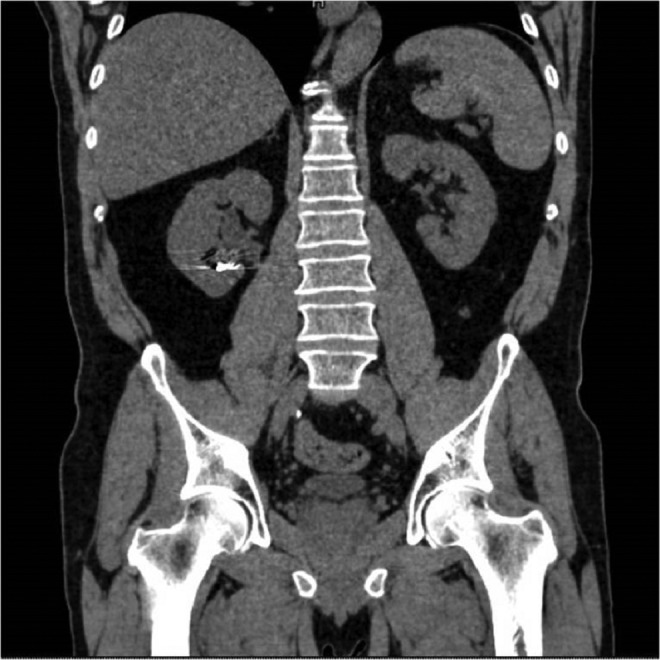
One-year postoperative CT scan showing nonresidual stone fragments, but only residual metal coils of previous SRAE.
